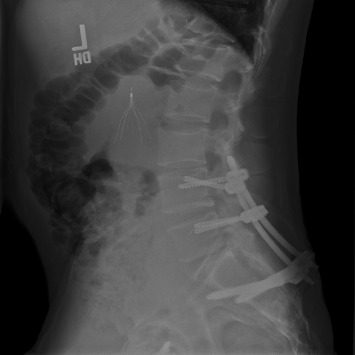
Postoperative X-ray in a patient who underwent open reduction and lumbopelvic fixation.
